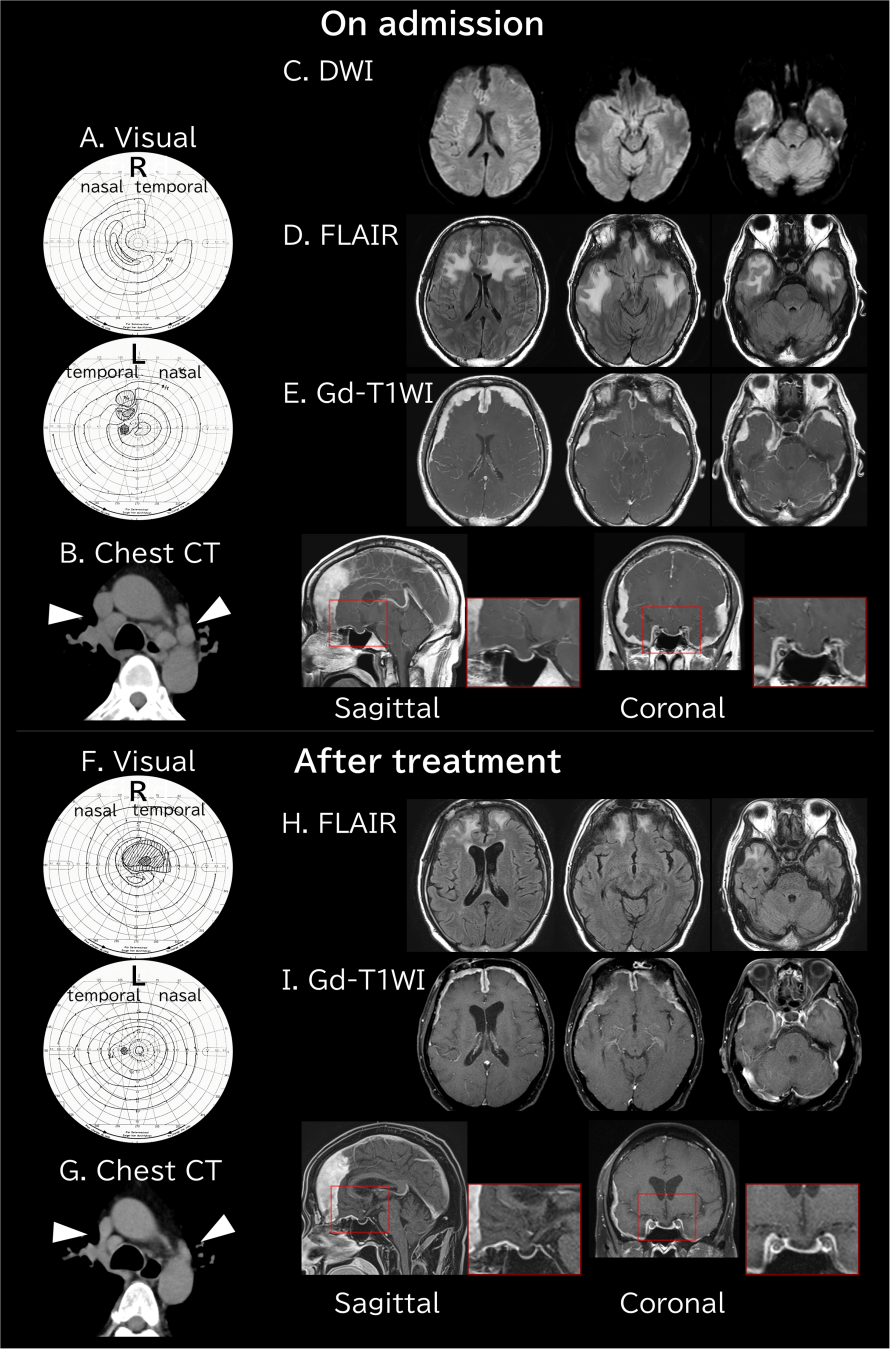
Visual field testing and radiological findings before and after treatment. (A) On admission, visual field testing showed loss of the upper temporal quadrant of the right visual field and a scotoma in the left visual field. (B) Chest CT revealed hilar lymphadenopathy (white arrowheads). (C) DWI showed no obvious diffusion restriction. (D) FLAIR images demonstrated widespread high-intensity signals in the anterior white matter. (E) Gd-T1WI revealed diffuse dural thickening extending into the frontal and temporal regions, with herniation of the rectus gyrus of the frontal lobe into the sella turcica. (F) Three months after steroid treatment, visual field testing showed recovery of the right peripheral visual field and reduction of the left scotoma. (G) Follow-up chest CT showed marked regression of the hilar lymphadenopathy. (H) The widespread high-intensity white matter signals had decreased, and the dural thickening had markedly improved. (I) Correspondingly, herniation of the rectus gyrus also showed improvement. CT, computed tomography; DWI, diffusion-weighted imaging; FLAIR, fluid-attenuated inversion recovery; Gd-T1WI, gadolinium-enhanced T1-weighted imaging; L, left; R, right.
